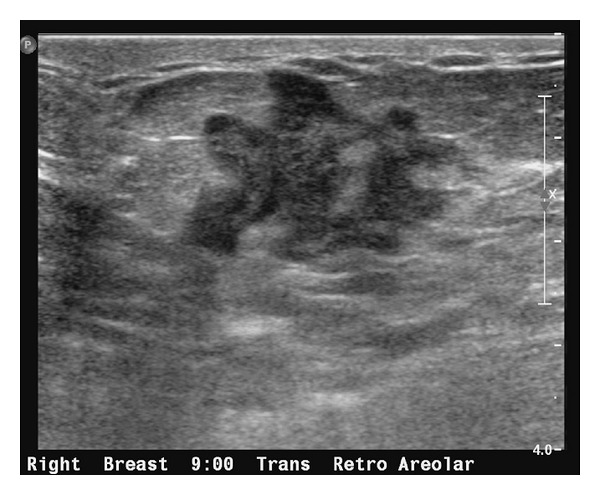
Focused ultrasound of the right breast in the retroareolar region better demonstrates the biopsy-proven invasive breast cancer.
